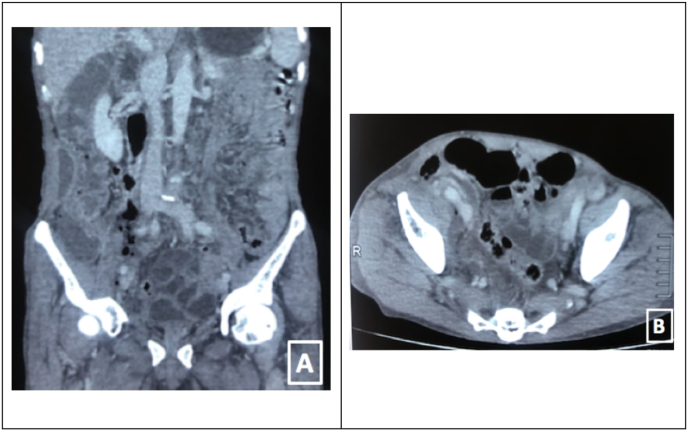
A. Coronal CT image showing gas tracking from the right perirectal area superiorly into the right retroperitoneum to the level of the medial and inferior right kidney. B. Axial CT image showing gas around the right rectum to the sacrum and ascending anterior along the right internal pelvic sidewall.
